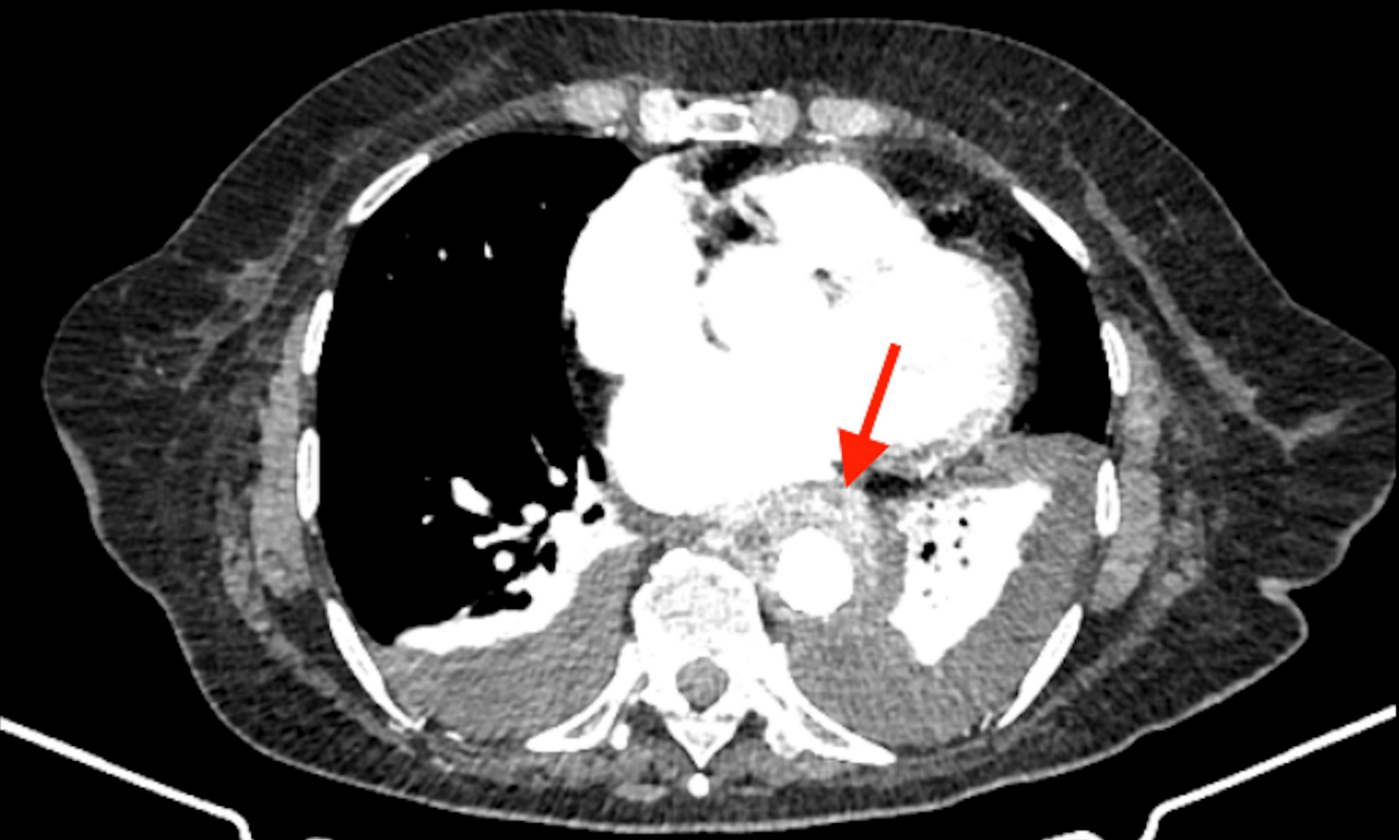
Computed tomography depiction of focal aortic aneurysm of the descending aorta (arrow).
